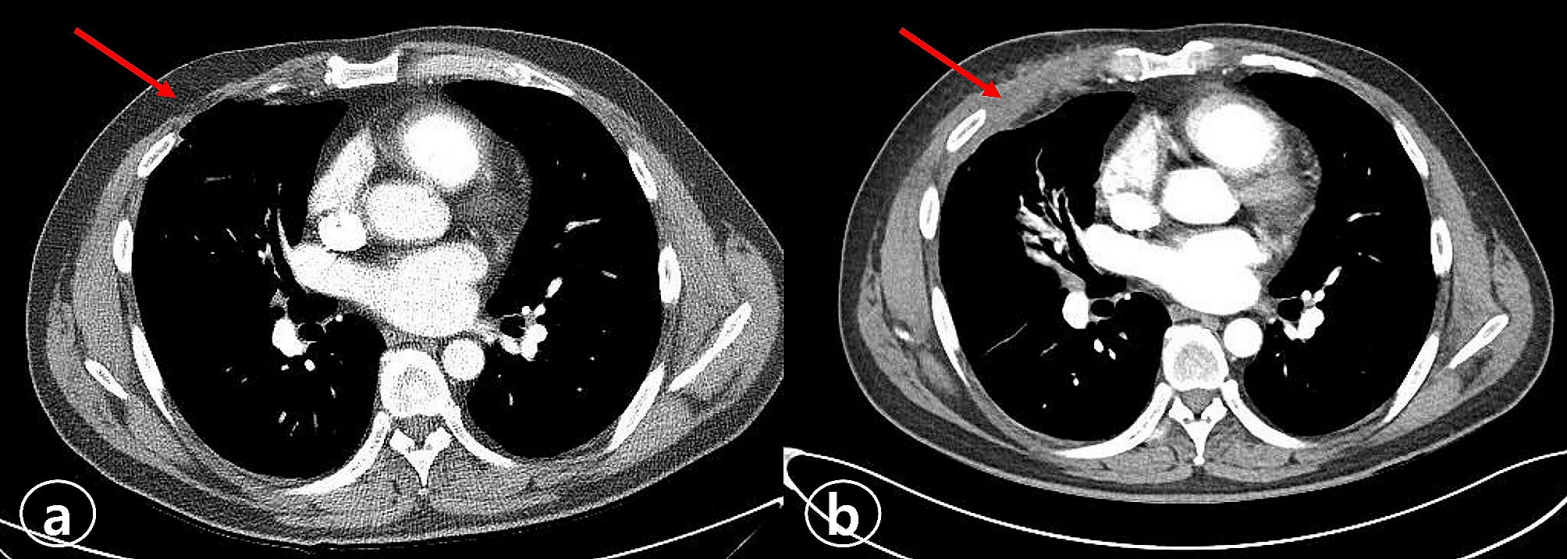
Preoperative and Postoperative CT scans (a) Preoperative chest computed tomography (CT) shows an intercostal lung hernia through the 4th intercostal space (arrow). (b) Postoperative chest CT demonstrates the disappearance of the intercostal lung herniation (arrow)
